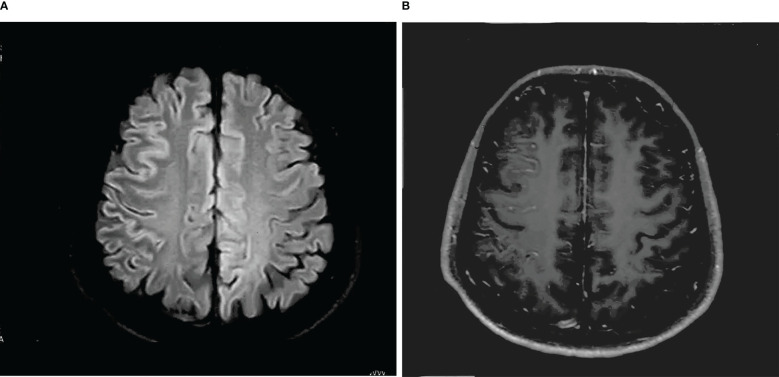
(A) T-2 FLAIR hyperintensity in the right frontal cortex, swelling of brain tissue, and shallowing of the cerebral sulcus. (B) Abnormal enhancement of the pia mater in the right frontal lobe.
